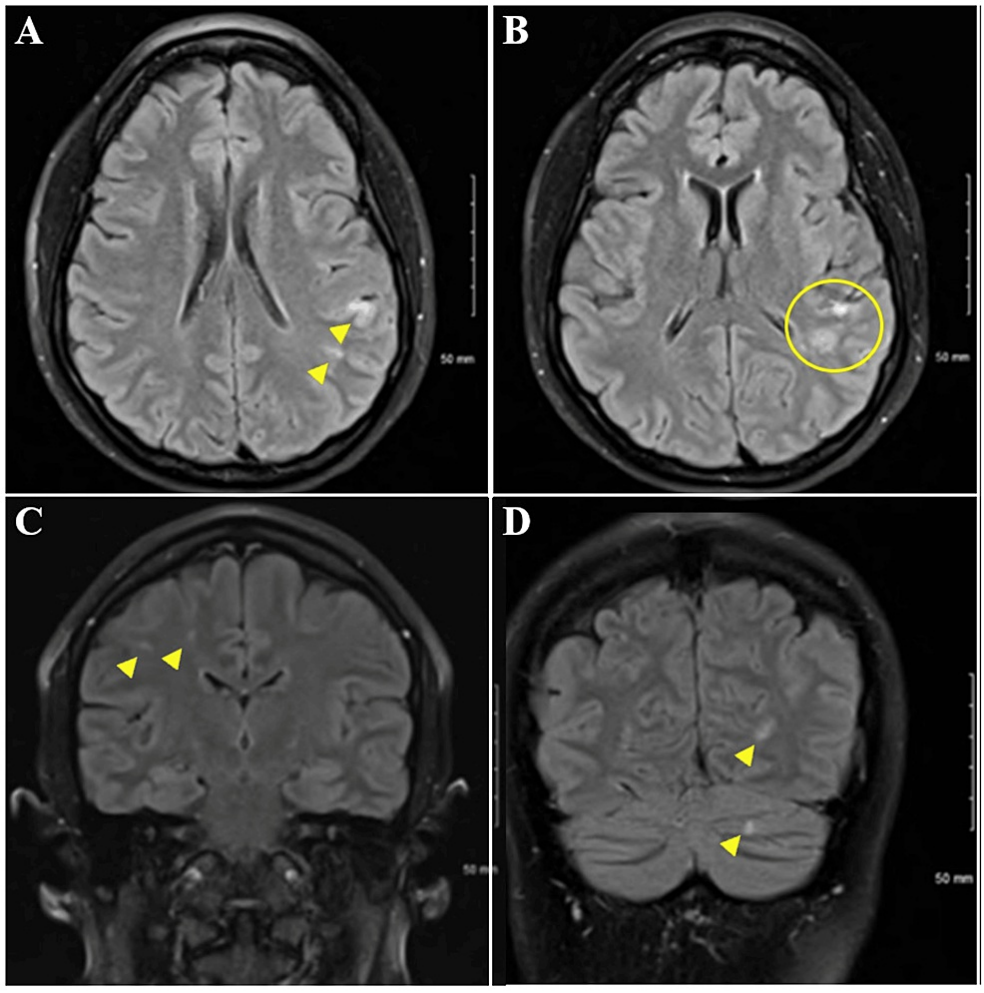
MRI brain, axial, and sagittal views Figure 1A: Acute infarction in the left parietal cortex and left parietotemporal lobe subcortical white matter (arrows) Figure 1B: Acute and subacute infarction in the left parietal cortex and left parietotemporal lobe subcortical white matter (circle) Figure 1C: Chronic small infarctions in the right parietal lobe Figure 1D: Chronic small infarction in the right posterior frontal subcortical white matter and left cerebellum.
